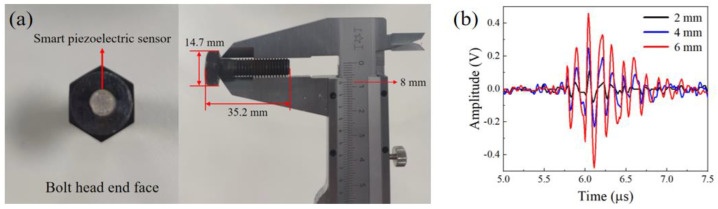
(a) M8 bolt with a smart piezoelectric sensor; (b) ultrasonic signal strength for different spot diameters.
